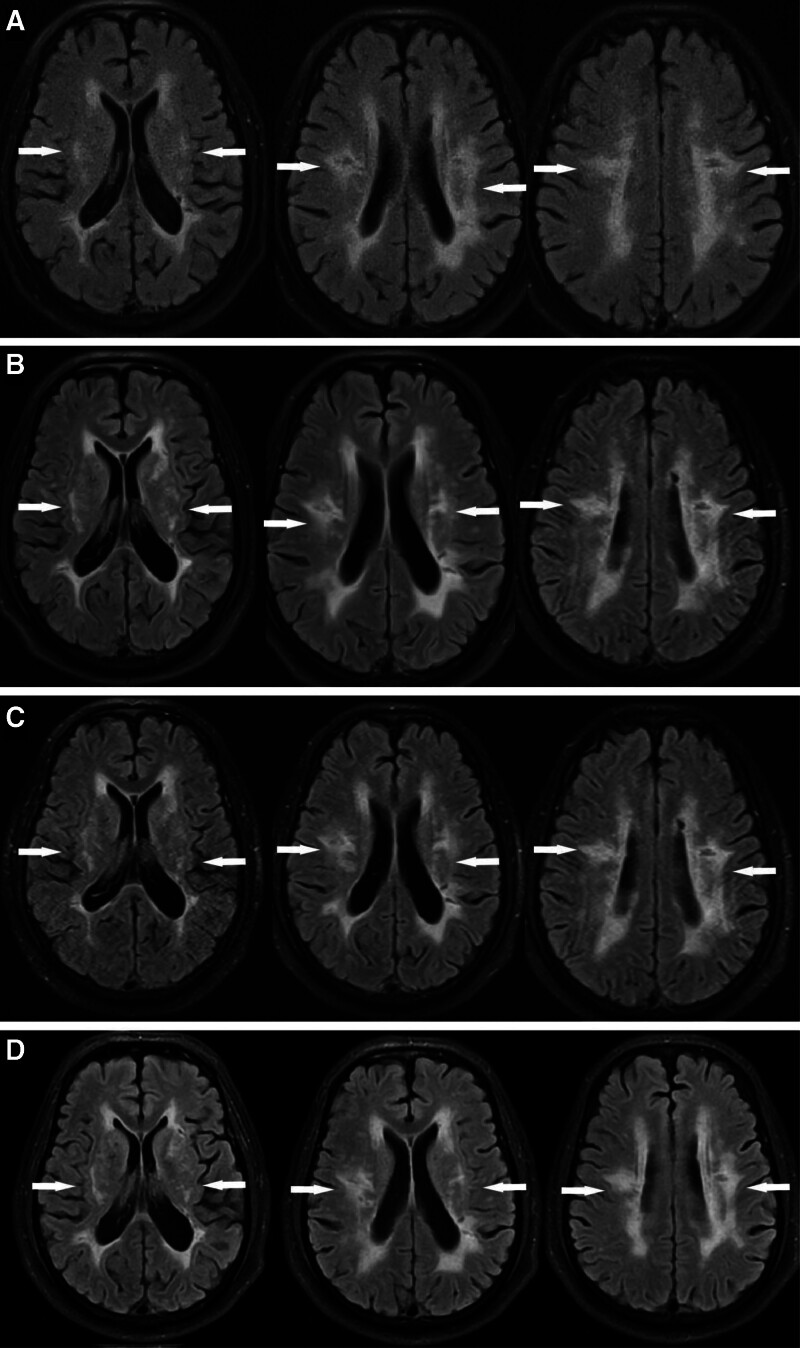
Brain MRI FLAIR order indicated high signals associated with the white matter near the bilateral ventricles. (A) 2021-10-02; (B) 2023-08-04; (C) 2024-02-21; (D) 2024-08-13. FLAIR = fluid-attenuated inversion recovery, MRI = magnetic resonance imaging.
